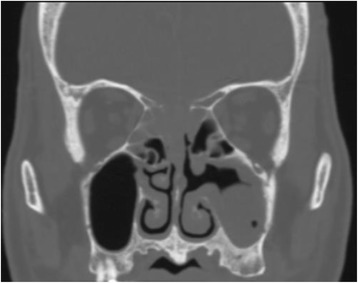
Coronal view of non-contrast-enhanced CT. A poorly marginated lesion with soft tissue density was observed in the superior nasal septum, invading the bone in the anterior cranial fossa. A mass with soft tissue density is apparent in the left maxillary sinus
